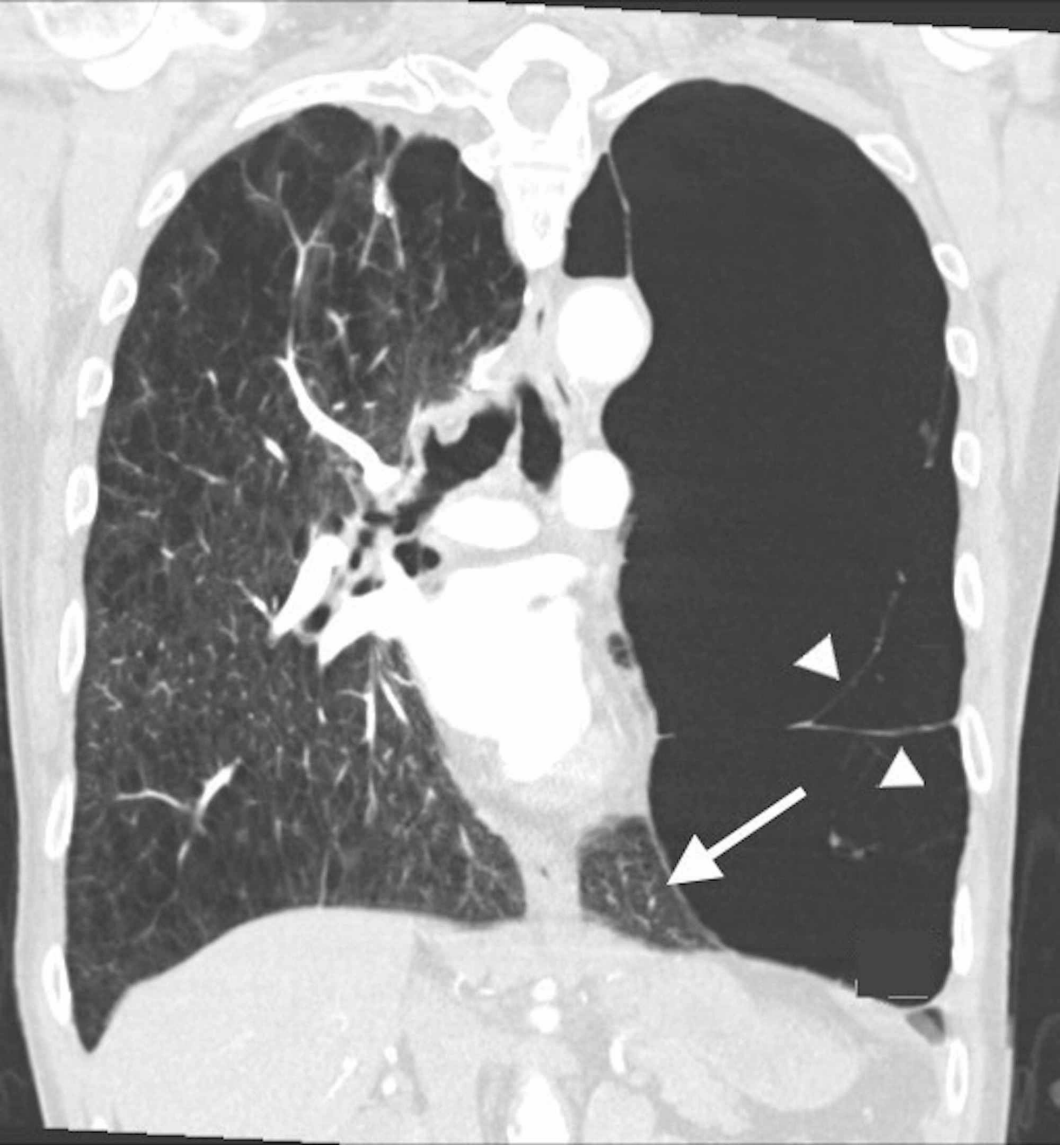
CT scan of the chest Coronal view showing a giant bulla with septae (arrowheads) involving entire left upper lobe of the lung, occupying more than two-third of the left hemithorax resulting in compression of left lower lobe of lung parenchyma (pointed with arrow).
